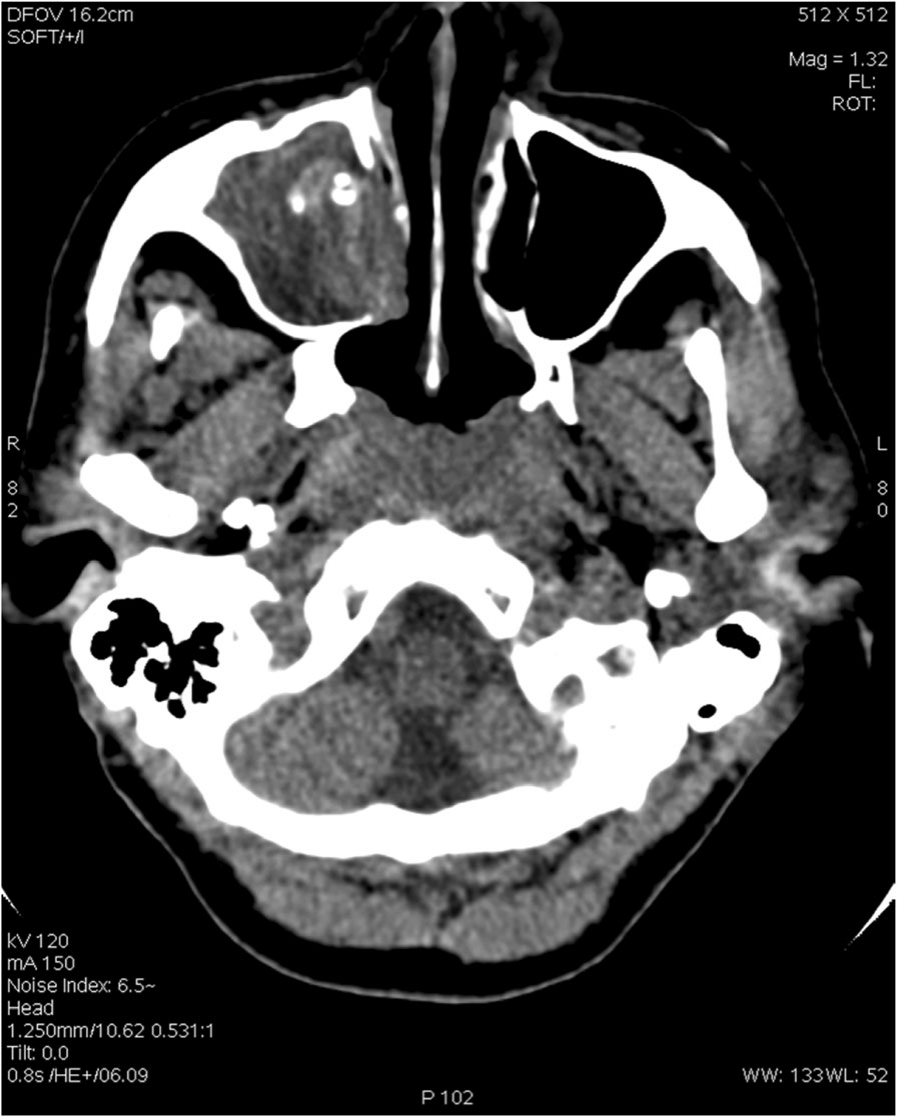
Representative Computerized-Tomography Scan (CT-Scan) of the sinuses showing complete opacification of right maxillary sinus with flocculent calcifications and an absence of bone erosion
